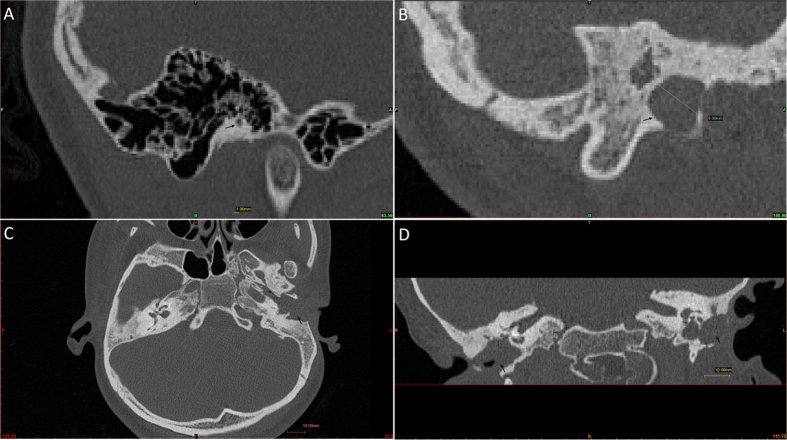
HRCT scans of CAS. (A) the right side stenotic EAC (black arrow) was 1 mm without cholesteatoma in an 8-year-old boy with CAS (sagittal view). (B–D) bilateral EAC cholesteatoma (black arrow) in a 17-year-old girl with CAS, the left side EAC was 9 mm (B, sagittal view; C, axial view; D, coronal view).
